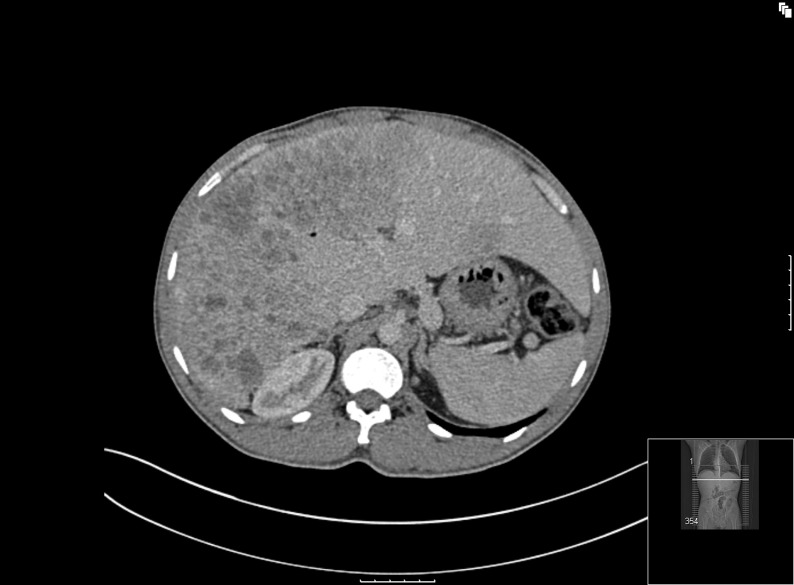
CT scan showing metastatic neuroendocrine tumour involving the liver and gallbladder.
